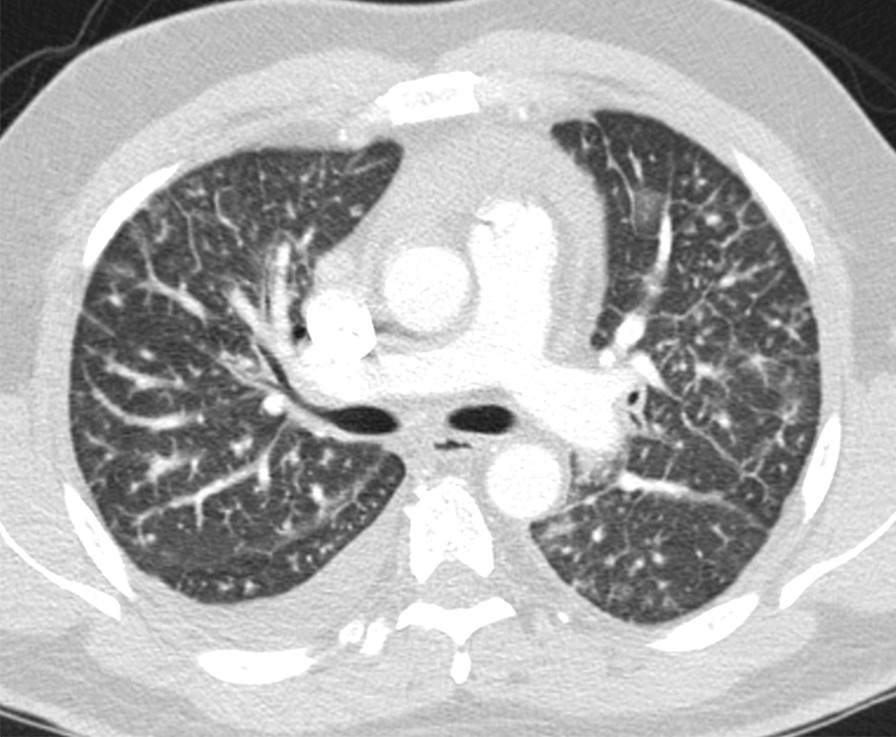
Chest computed tomography with lung window reveals diffuse bilateral septal thickening, compatible with venolymphatic congestion/pulmonary edema
